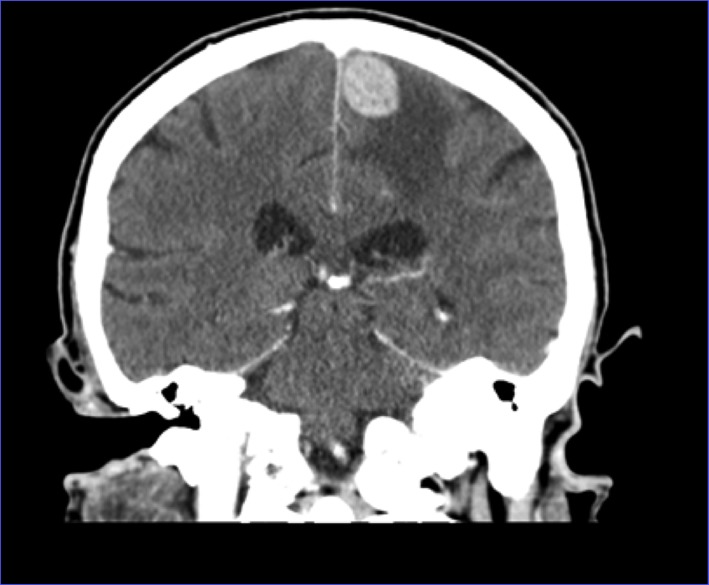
CT of head coronal view showing left frontal mass with surrounding edema.
